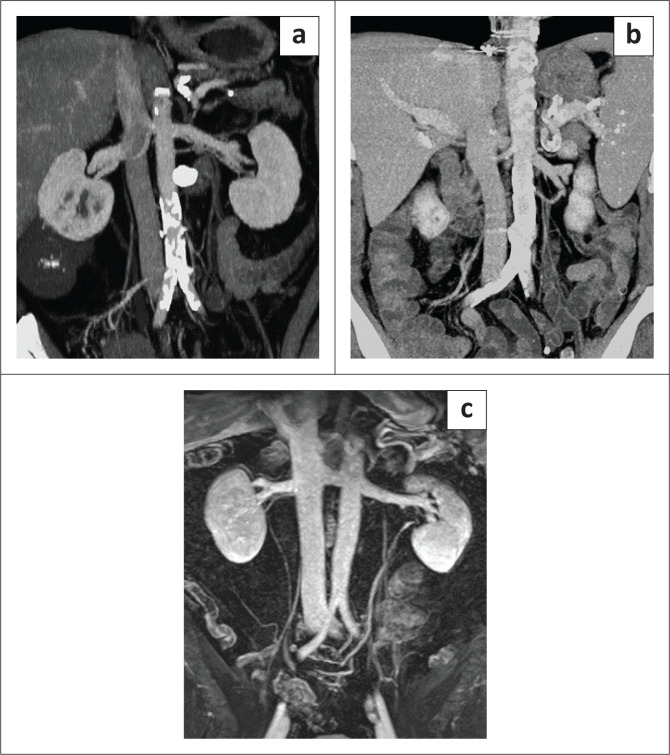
(a) Coronal CT abdomen in the portal venous phase showing denser contrast mixing into the inferior vena cava (IVC). (b) Coronal CT abdomen image acquired at 90 s showing homogeneous enhancement of the IVC. (c) Indirect contrast-enhanced magnetic resonance venography image demonstrating a normal IVC to the right of the aorta.
